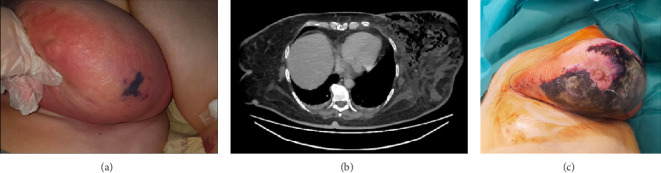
(a) Initial presentation at the ER showing swelling, redness, and a small necrotic area in the inferior internal quadrant of the breast. (b) Thoracic CT scan revealing extensive subcutaneous emphysema. (c) Progression of necrosis affecting the entire breast.
